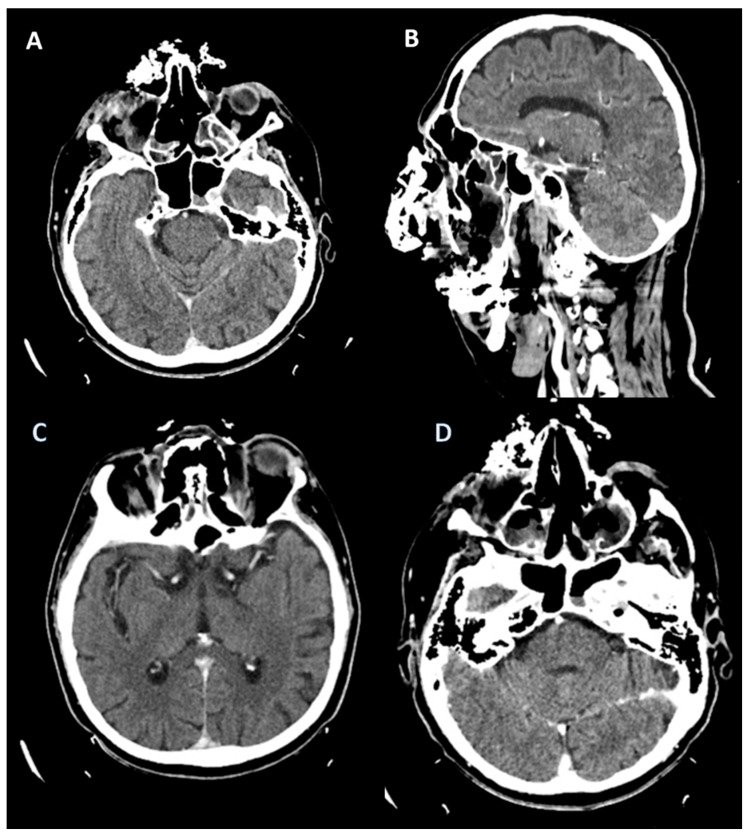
Cranial CT (scale bar equals 1.25 mm). (A,B) The image of the coronal computed tomography shows thickening of the mucosa and homogeneously walled-off collections with air inclusions, localized in the left sphenoidal sinus, bilateral frontal maxillary sinuses, and ethmoidal cells, post-necrotic bone structure changes with the interruption of the bone cortex, and multiple osteolytic lesions in the bilateral fronto–ethmoido–maxillary regions, as well as in the nasal septum and nasal bones. (C,D) Right eyeball with reduced dimensions and altered structure with a shrunken appearance.
